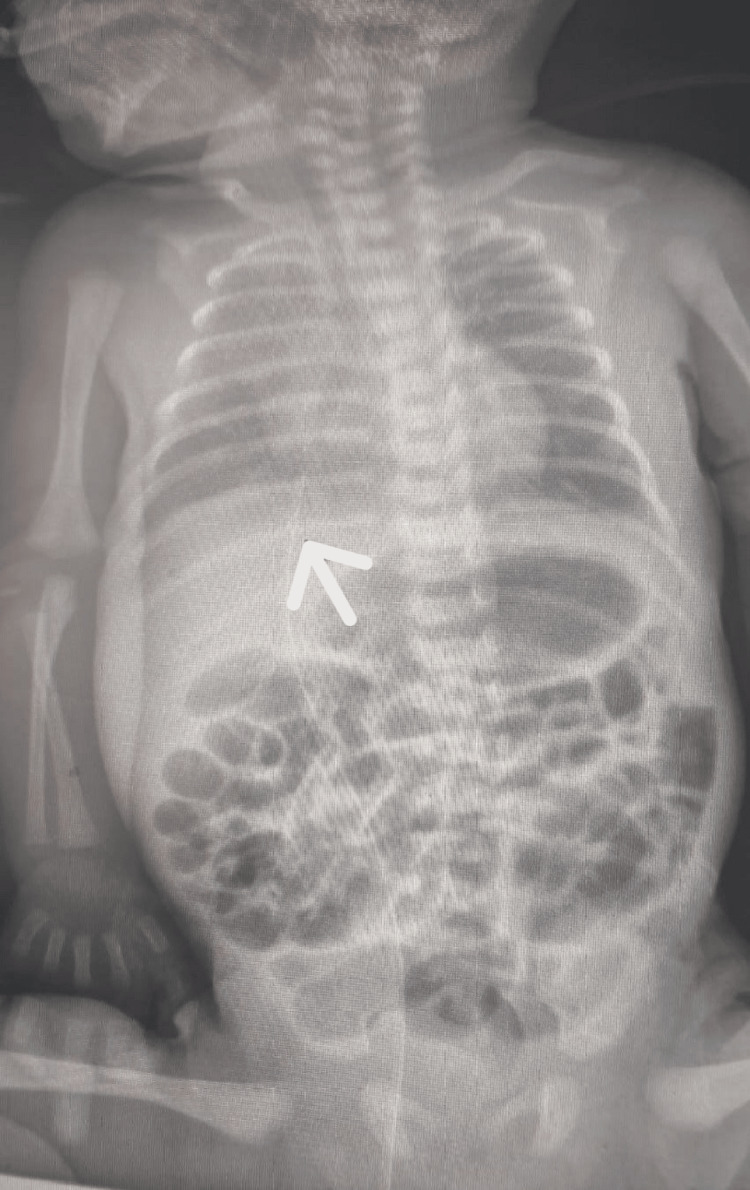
X-ray showing the unrecognized malposition of the umbilical venous catheter
